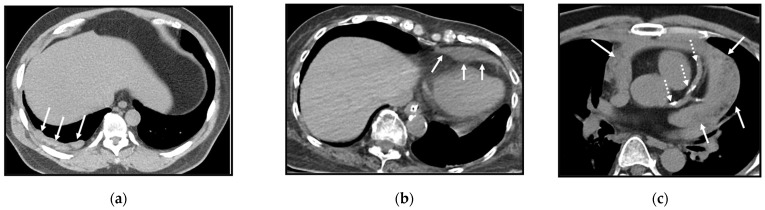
The incidental findings of the pleura and pericardium on a low-dose computed tomograph (LDCT). The LDCT shows (a) focal and irregular thickening of the right lower pleura (arrows) of a 46-year-old male; (b) asymmetrical thickening of the lower pericardium measuring 1.6 cm in thickness (arrows) of an 80-year-old female, where the normal pericardium thickness should be less than 4 mm; and (c) pericardial effusion measuring up to 3.1 cm in thickness (arrows) of a 39-year-old male. The incidental finding of left coronary artery calcification was also noted (dashed arrows).
